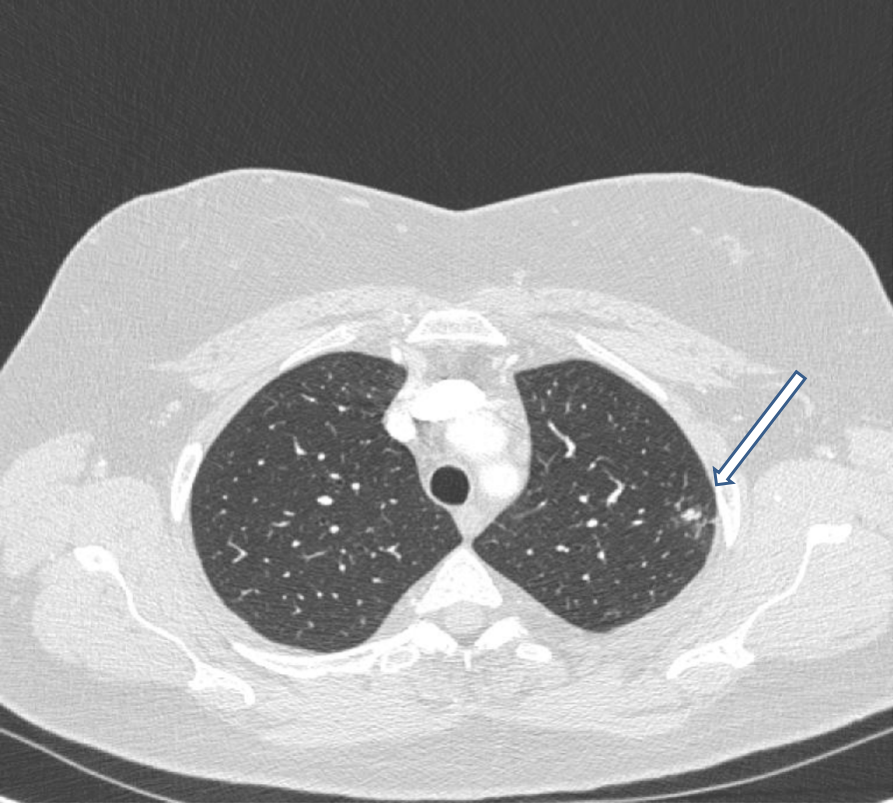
CT of the chest in 2012 showing a sub-pleural fistula in the left upper lobe (arrow).
